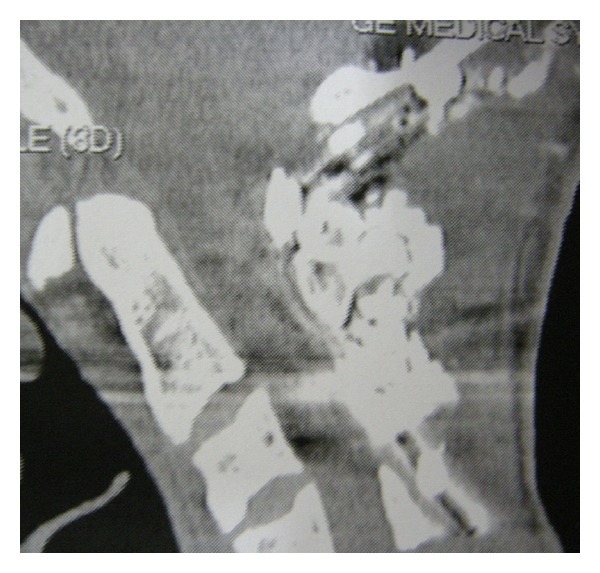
Computed tomography scan showing an occipito-cervical stable fusion at the 3-year followup.
